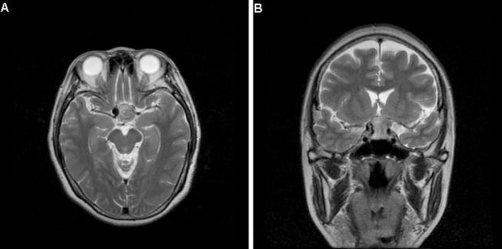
Axial (A) and Coronal (B) view of T2-weighted MRI showing a round flow-void mass in the right supraclinoid ICA associated with a pituitary macroadenoma.
